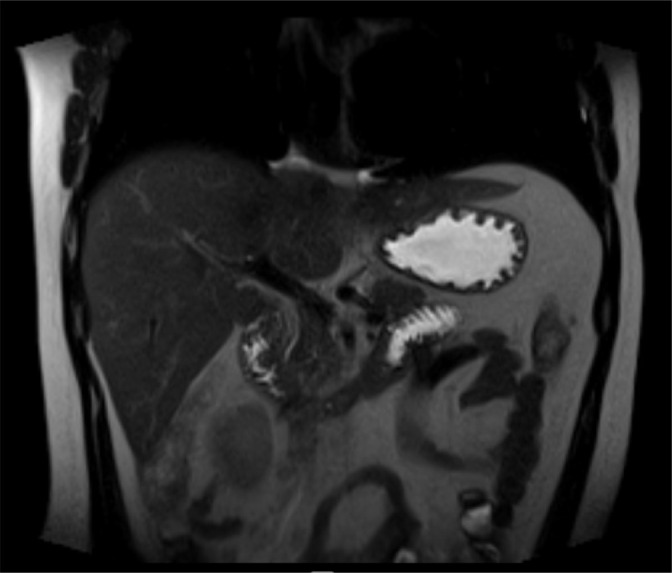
Magnetic resonance image suggesting *pancreas divisum.*
